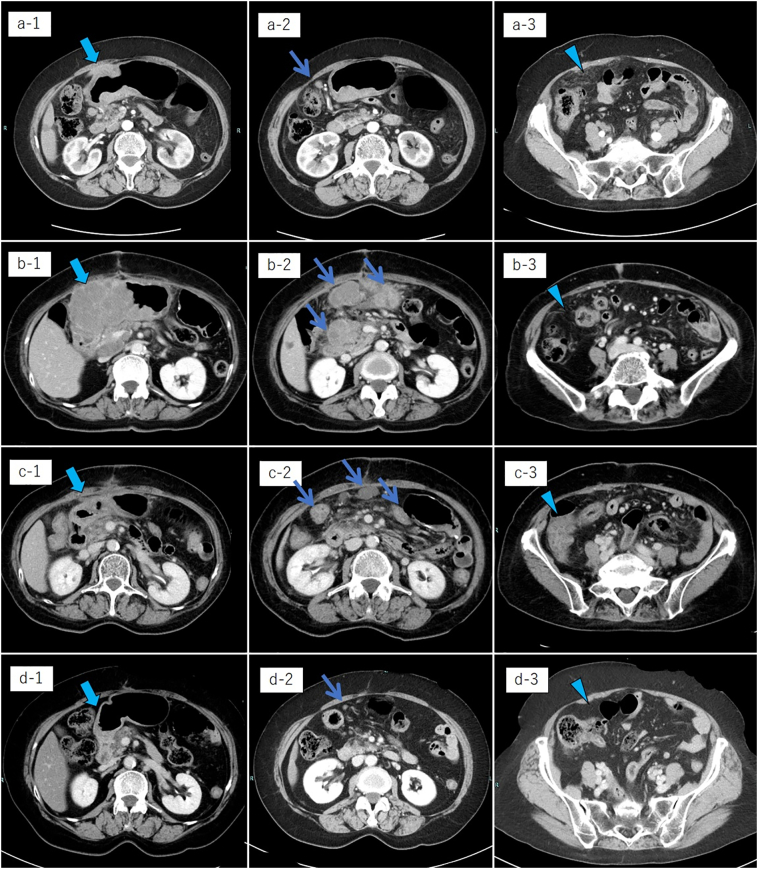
Contrast-enhanced CT examination showed the transition of the therapeutic effect in the primary lesion in the body of the stomach (thick arrow), lymph nodes (thin arrow), and peritoneal dissemination (arrow head).
